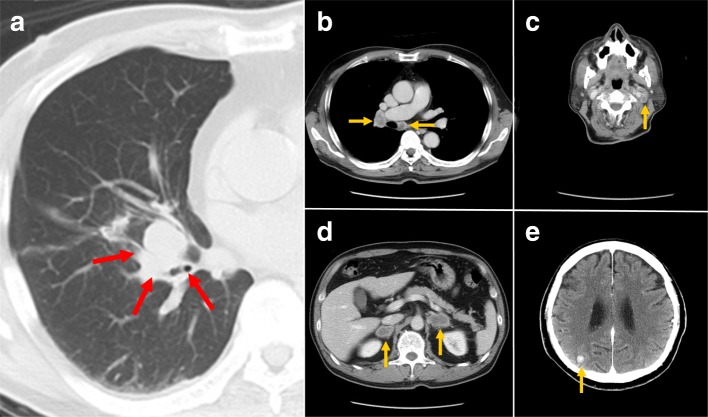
Far-advanced lung cancer on CT survey. a A primary lung tumor (red arrows) was located in the right middle lobe. Metastatic nodules (yellow arrows) were noted in the b lymph nodes at the hilum of the right lung and lymph nodes at the bifurcation of the trachea and c lymph nodes at the left side of the neck, d bilateral adrenal glands, and e brain
